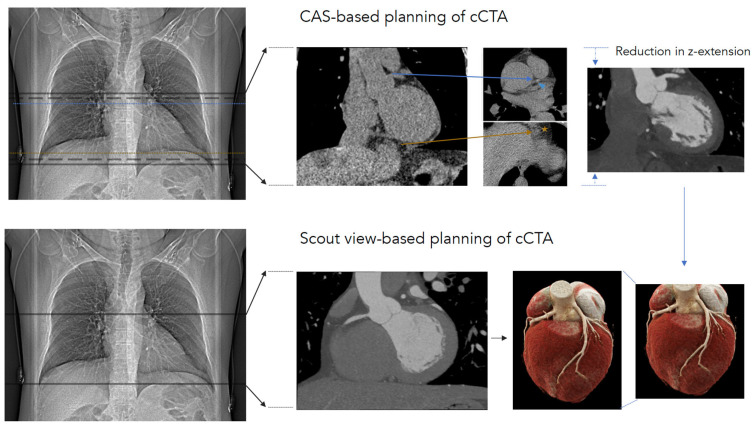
To obtain a complete depiction of the coronary tree, either calcium-scoring CT-based or scout-view-based planning is feasible. With regard to calcium-scoring CT-based planning (upper row), a calcium-scoring CT scan was planned in anterioposterior (AP) scout view presenting an image 1 cm below the carina and 1 cm below the cardiac apex (solid lines on AP scout view). In the calcium-scoring images, the most cranial part of the coronary tree (blue arrowhead) and the cardiac apex (orange star) are depicted (dotted lines in AP-scout view). CT coronary angiography scan length was set at 1 cm with respect to the cranial and caudal regions of those landmarks (dashed lines on AP-scout view). In scout view-based coronary CT angiography planning (lower row), the scan length was planned based on the scout view, with 1 cm below the carina and 1 cm below the cardiac apex defined as landmarks. The calcium-scoring CT scan itself can be omitted in such an approach.
